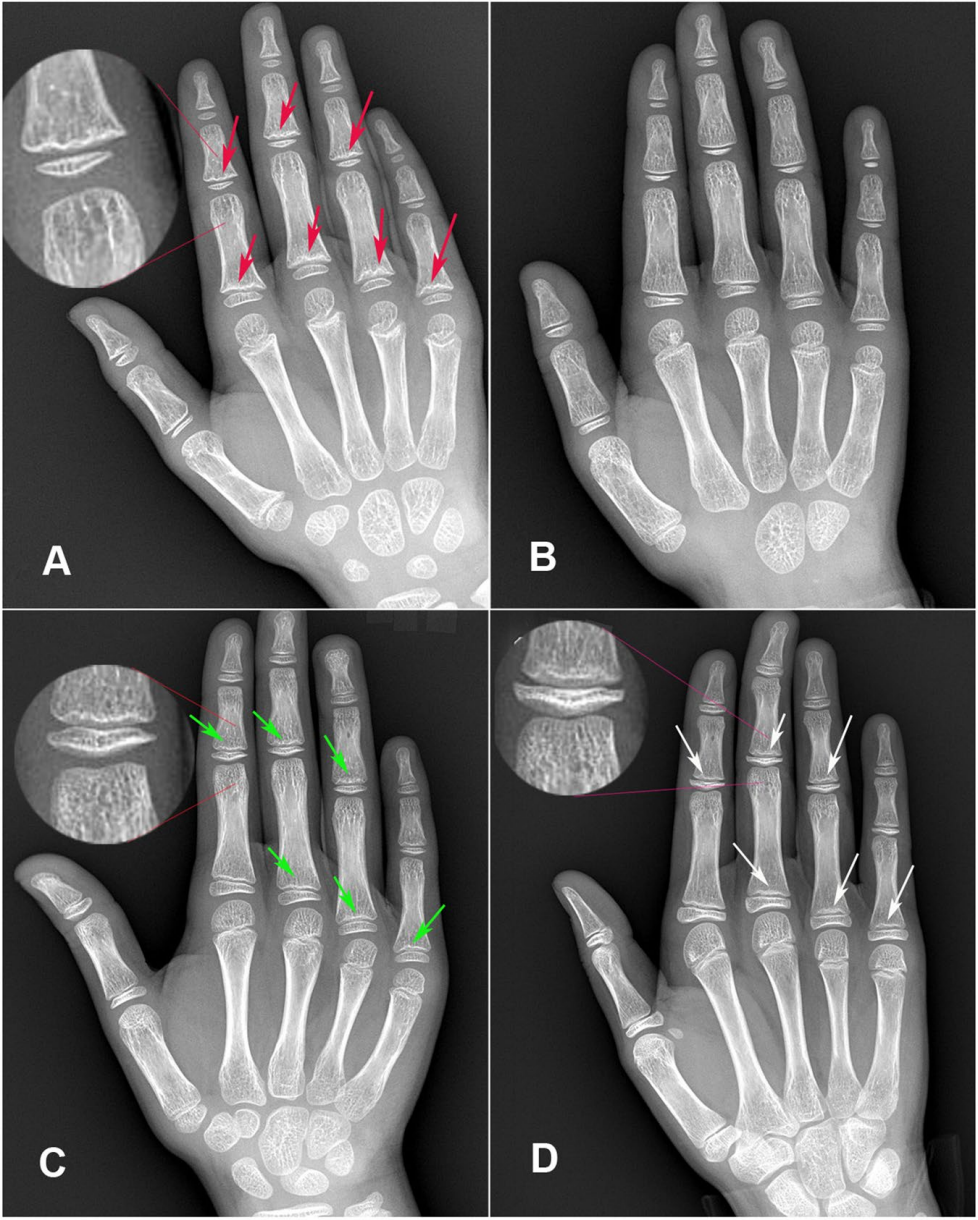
(A) Is a radiological image of the right hand with KBD-positive X-ray signs in an 8-year-old boy: some metaphyseal hardening signs, waviness or serration changes and small depressions (red arrows) are found in the zones of provisional metaphyseal calcification in the fingers (including index finger, middle finger and ring finger). (B) Is a radiological image of the right hand of a 7-year-old healthy boy: all metaphyses, epiphyses and diaphyses were glabrate, uniform, sinuous and non-destructive. The carpals were not completely formed. (C) Is a radiological image of the right hand of an 11-year-old girl with CRME in the equal-diameter period: the diameter of metaphysis and epiphysis are almost equal. Although it belongs to the normal development periods before complete closure of metaphysis-epiphysis (CCME), its imaging signs are similar to KBD-positive X-ray signs. Index finger, middle finger, ring finger and little finger show metaphyses serrated, wavy (green arrows). (D) Is a radiological image of the right hand of a 10-year-old girl with CRME in the ultra-diameter period: The diameter of epiphysis is longer than that of metaphysis. It occurs after the equal-diameter period normally and the features are similar to KBD-positive X-ray signs. Some metaphyseal waviness, serration and irregularly depressions are found in many fingers (white arrows).
